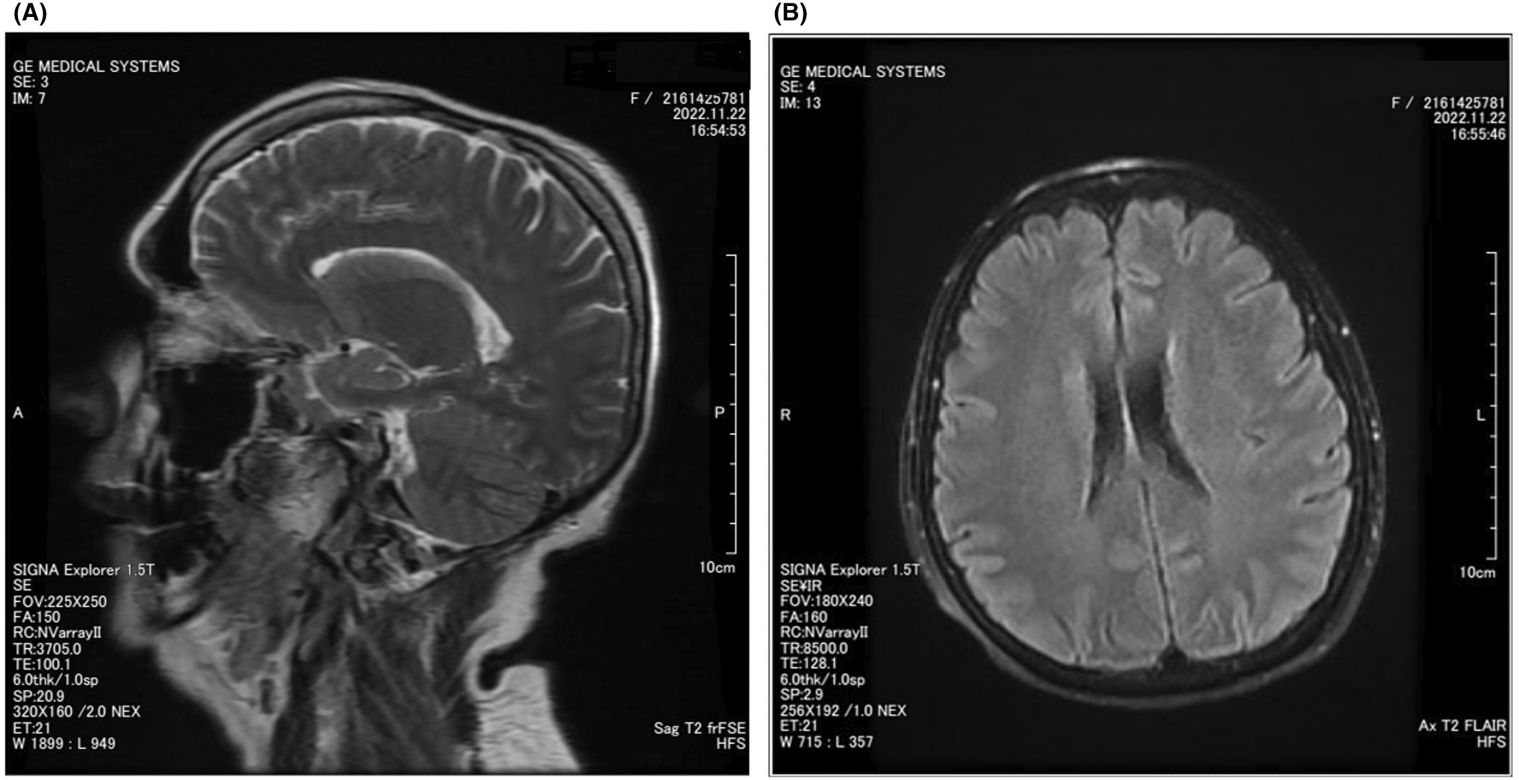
(A, B) Patient's brain MRI.
